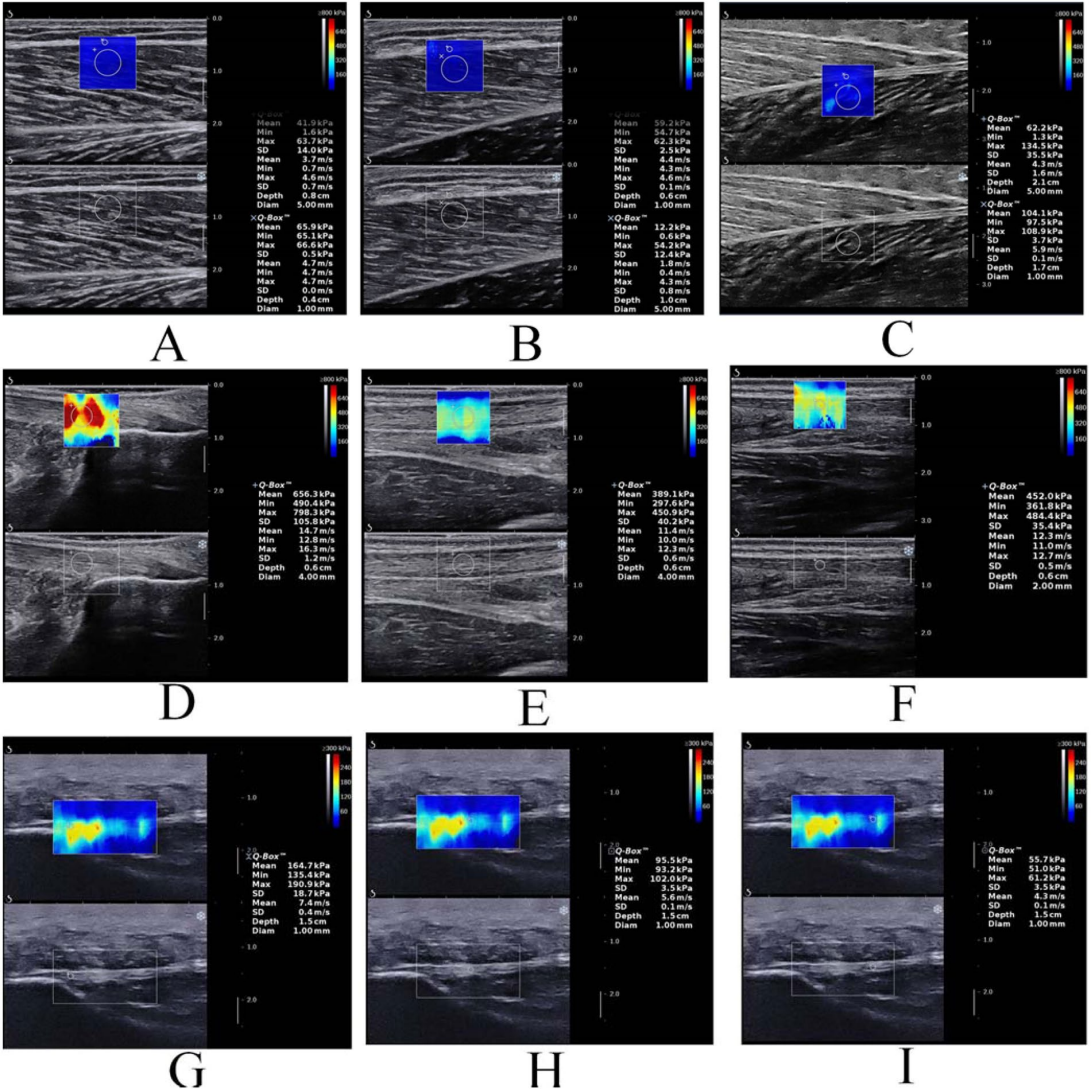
A longitudinal SWE sonogram of the MG, LG, SOL, AT and PF showing the measurements of the shear modulus at different regions (from A to I). The color scale for the muscle-tendon-fascia elasticity is shown in the upper images. The longitudinal grayscale sonograms of the muscle-tendon-fascia are shown in the bottom images. The Q-Box is shown on the right. (A) The shear modulus values of the LG; (B) the shear modulus values of the MG; (C) the shear modulus values of the SOL; (D–F) the shear modulus values of AT0cm, AT3cm and AT6cm, respectively; (G–I) the shear modulus values of PF-pro, PF-mid and PF-dis, respectively.
